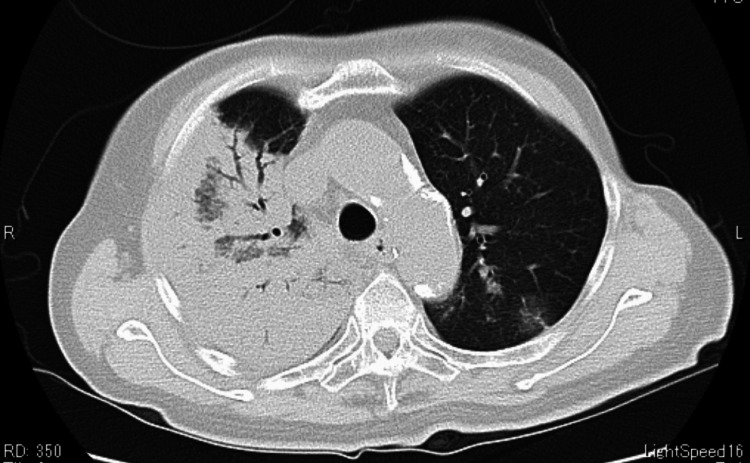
Chest CT performed 48 hours after admission showed severe pneumonia in the right lobe
